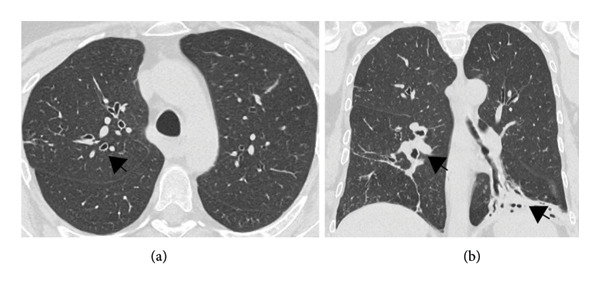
High‐resolution computed tomography (HRCT) with axial (a) and coronal (b) views showing bronchiectasis and cystic changes in consolidations (see black arrows).
